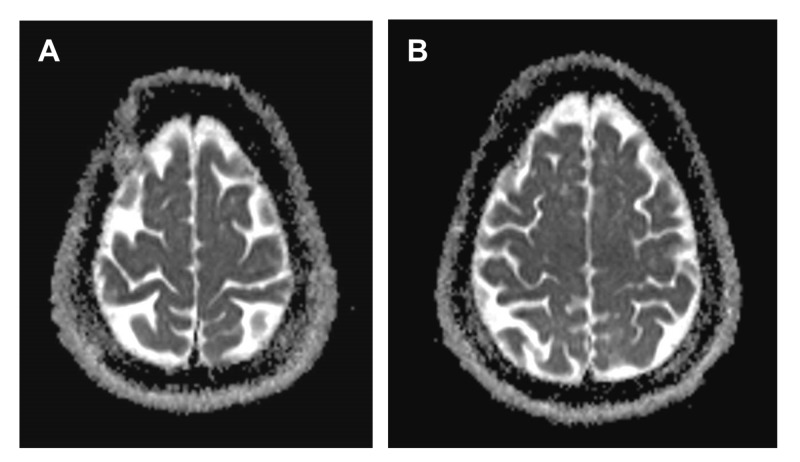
(A and B) MRI showing normal ADC in the cortical regions supplied by anterior circulation. MRI, magnetic resonance imaging; ADC, apparent diffusion coefficient
